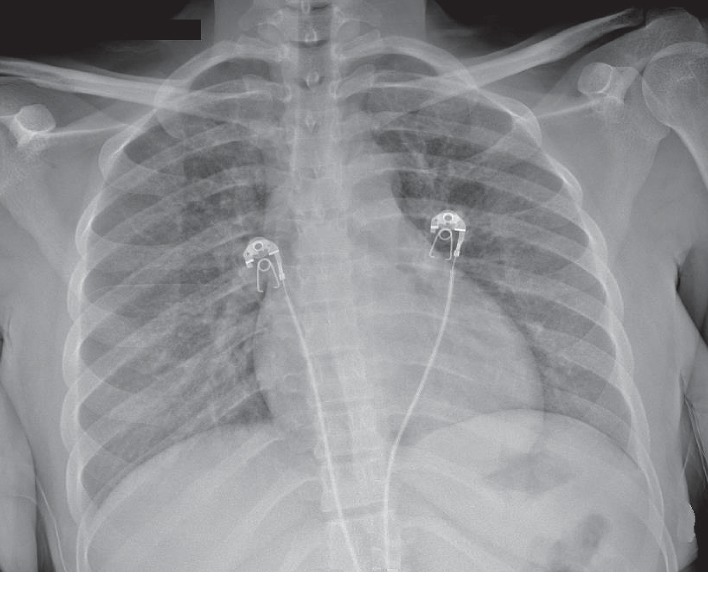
Chest radiograph showed bilateral homogeneous ground-glass opacity.
